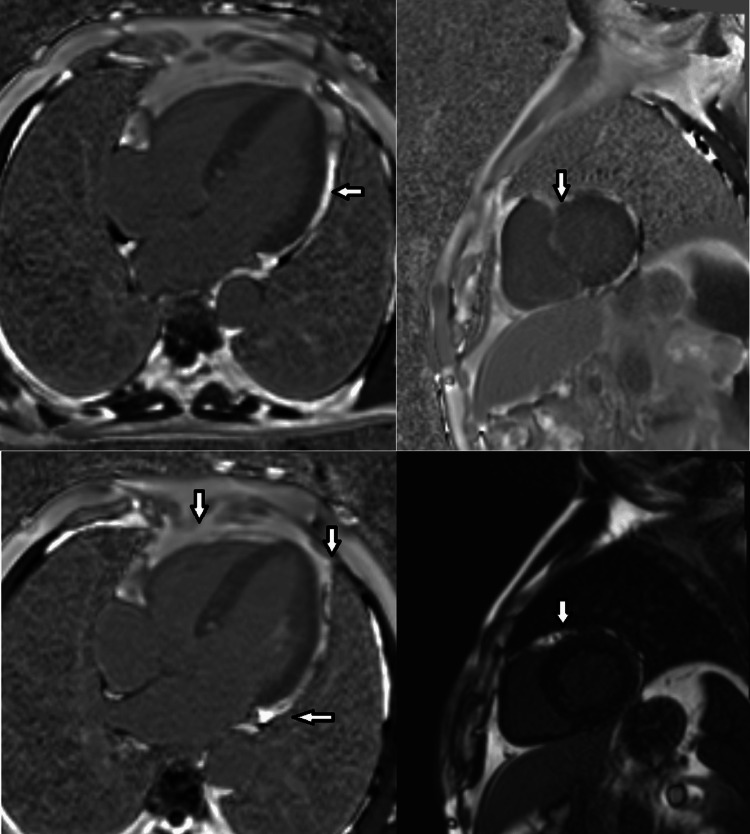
CMR showing increased wall thickness, multiple regions displaying heightened T2 signal intensity (regional edema), and increased ratio of early gadolinium enhancement in the myocardium (white arrows) CMR: cardiac magnetic resonance imaging
